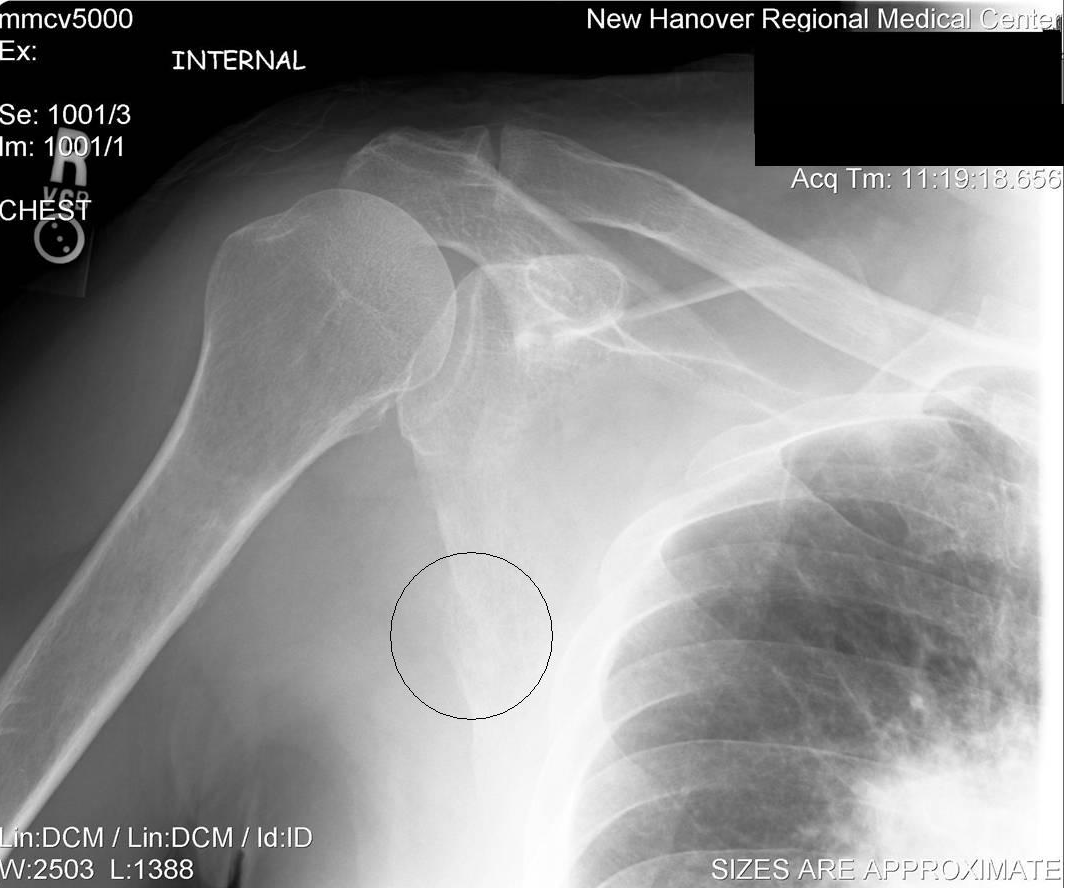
AP radiograph of the right scapula reveals a focal indistinct lucency and lytic destruction of the lateral scapular cortical margin.
